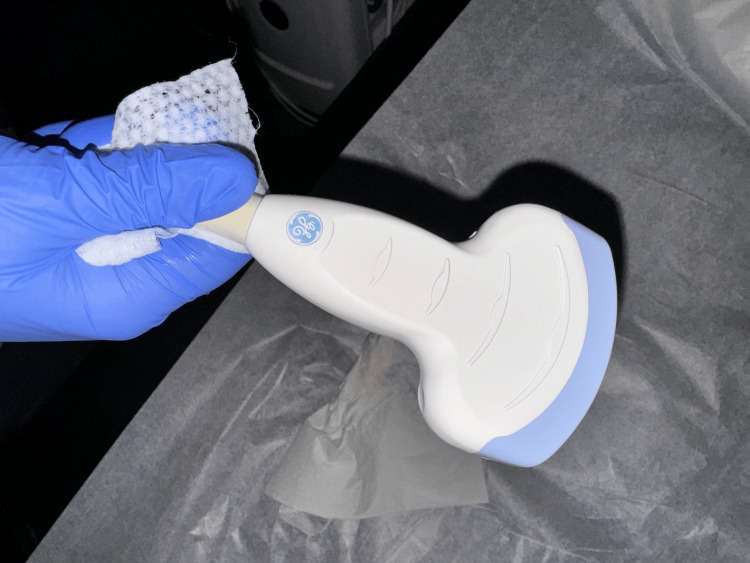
Curvilinear probe Image adapted from: Harrison Keely. Ultrasound probe. Available from https://openverse.org/image/64744439-6b47-4e29-8bdf-f8a3bec414c3?q=ultrasound+probe&p=9. Licensed under CC BY 4.0
